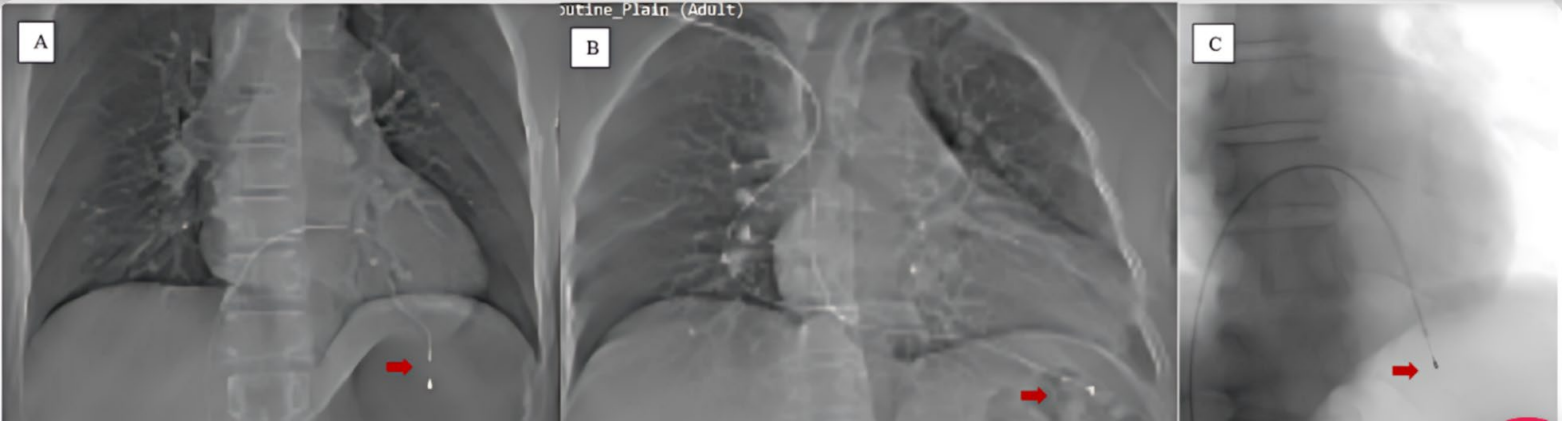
chest X-ray (A, B) and fluoroscopy (C) images showing temporary lead perforation (red arrows) in three different patients
